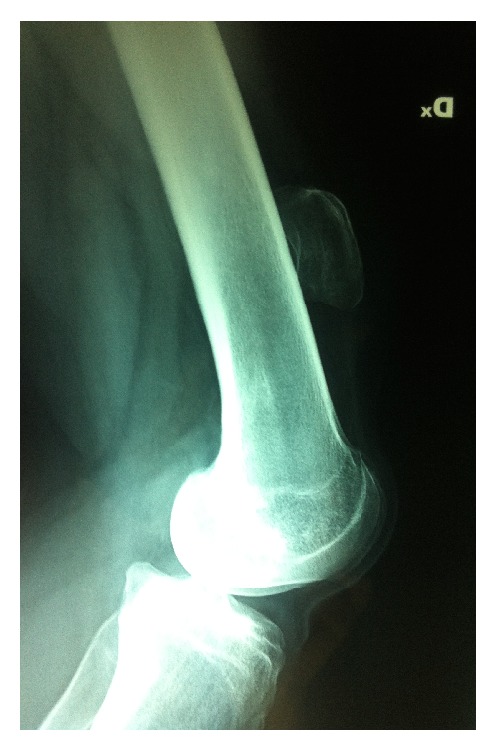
The LL X-ray of the knee showed no fractures, but an evident proximal dislocation of the patella and some calcific deposits on its inferior pole.
